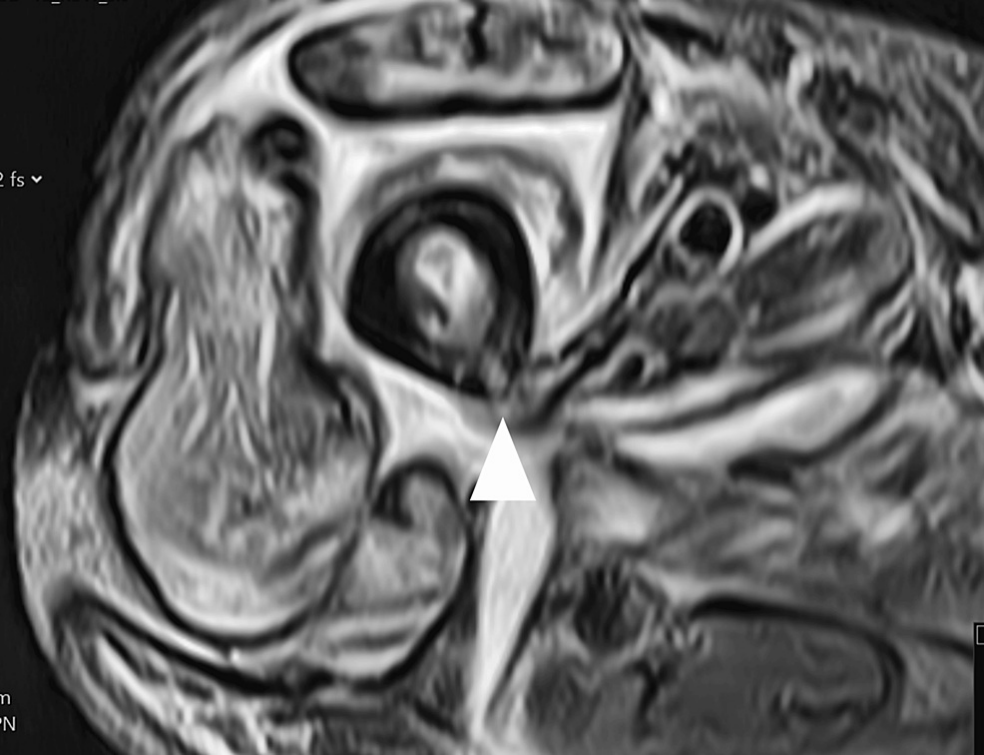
A T2-weighted axial slice of the right thigh The report noted hyperintensity in the anterior compartment where the majority of the fluid was. Highlighted are osteomyelitis-related changes. The high T2 signal in the bone marrow represents femoral canal necrosis.
